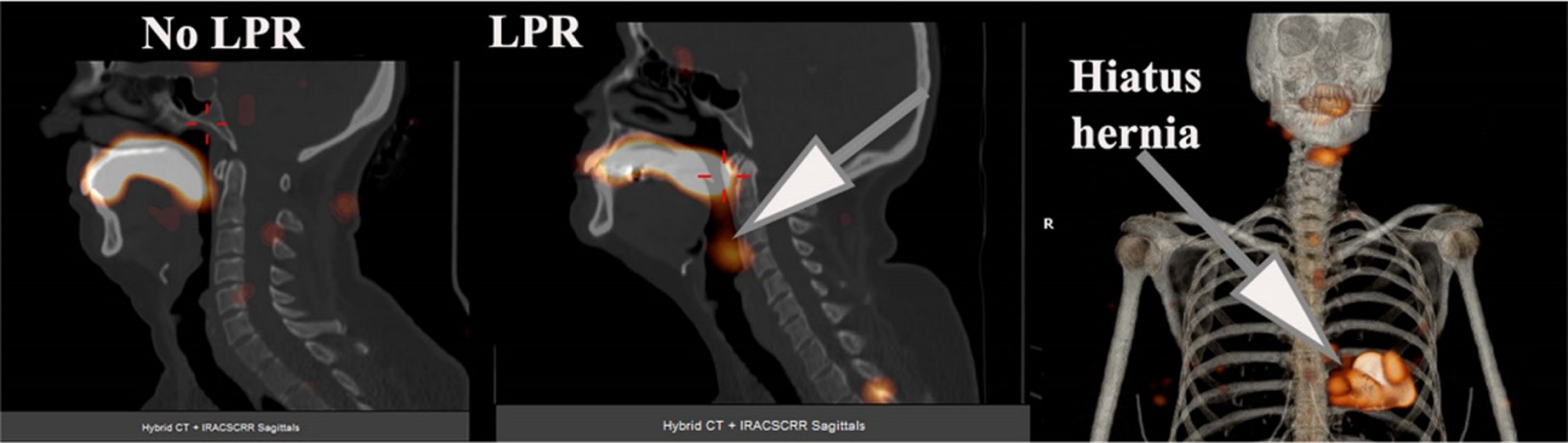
SPECT/CT of sagittal fused images showing a patient with no LPR and the typical pattern of LPR (arrow). The third images is the MIP of the thorax showing a hiatus hernia. LPR, laryngopharyngeal reflux; MIP, maximum intensity projection; SPECT/CT, single‐photon emission computed tomography/computed tomography.
